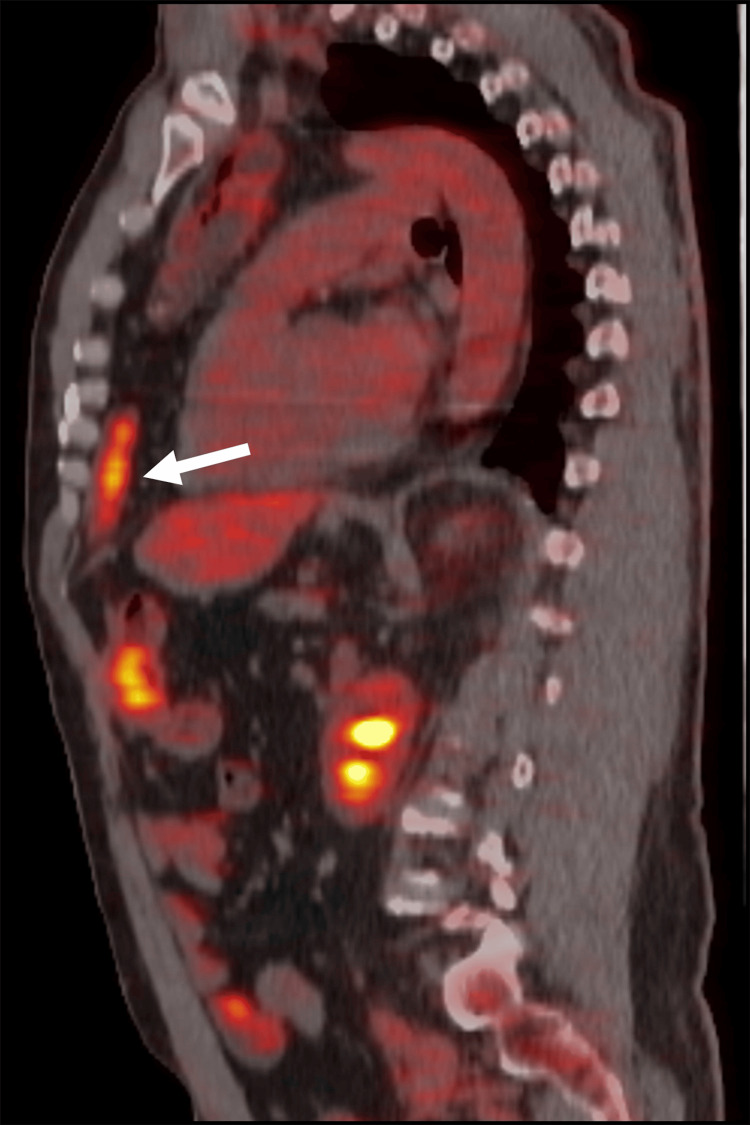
FDG-avid lesion in the distal colonic interposition. 18-fluorodeoxyglucose (FDG) PET/CT demonstrates focal hypermetabolism (arrow) in the distal colonic interposition, consistent with tumor involvement.
